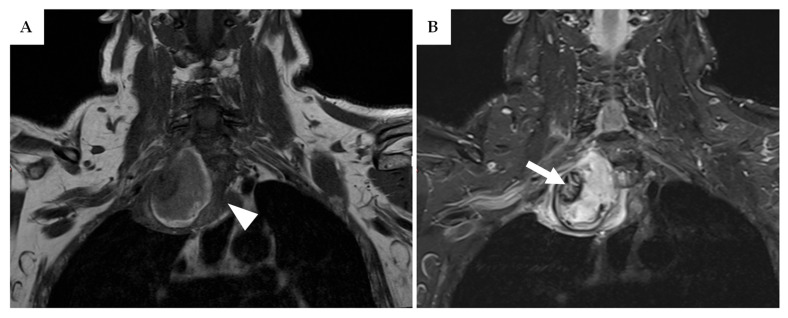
(A) T1-weighted TSE MRI of patient 1, showing a coronal view of the suspected lesion involving the right brachial plexus. The presence of extensive perilesional edema (white arrowhead) and a large tumour diameter of 70 mm in a patient with NF1 is highly suggestive of malignant transformation of a plexiform neurofibroma (PN). (B) T2-weighted STIR MRI of the same coronal slice as in image (A). A T1- and T2-hypointense nodule (white arrow) is visible in the mid-right portion of the tumour, which was later identified as a necrotic focus indicative of malignant transformation.
